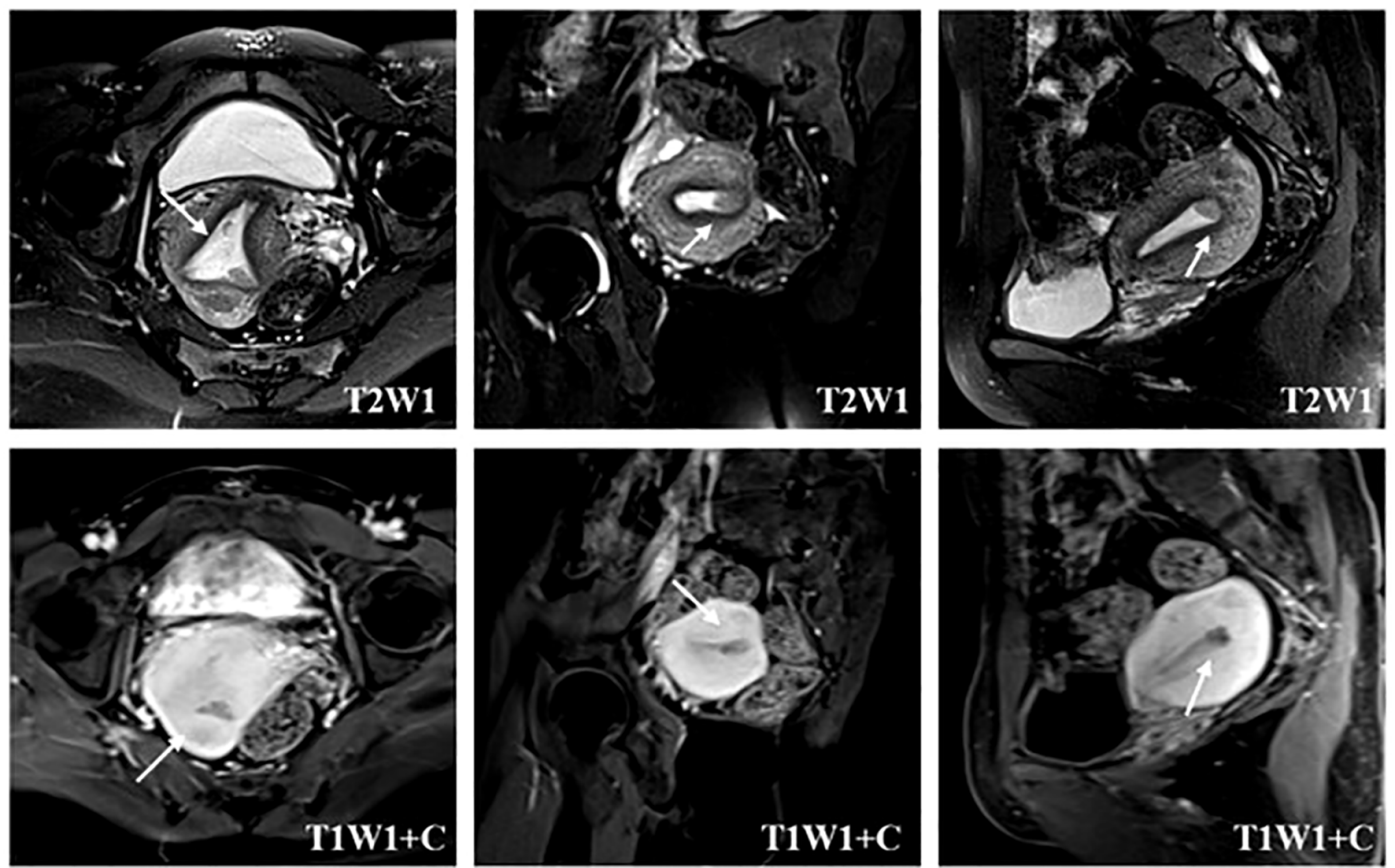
Localization of suspected myometrial invasion on MRI. The T2-weighted image reveals the heterogeneous signal intensity of the endometrial–myometrial junctional zone (arrow) in the lower left uterine horn. The contrast-enhanced T1-weighted image depicts an interrupted enhancing line (arrow) under the endometrium, suggesting myometrial invasion.
